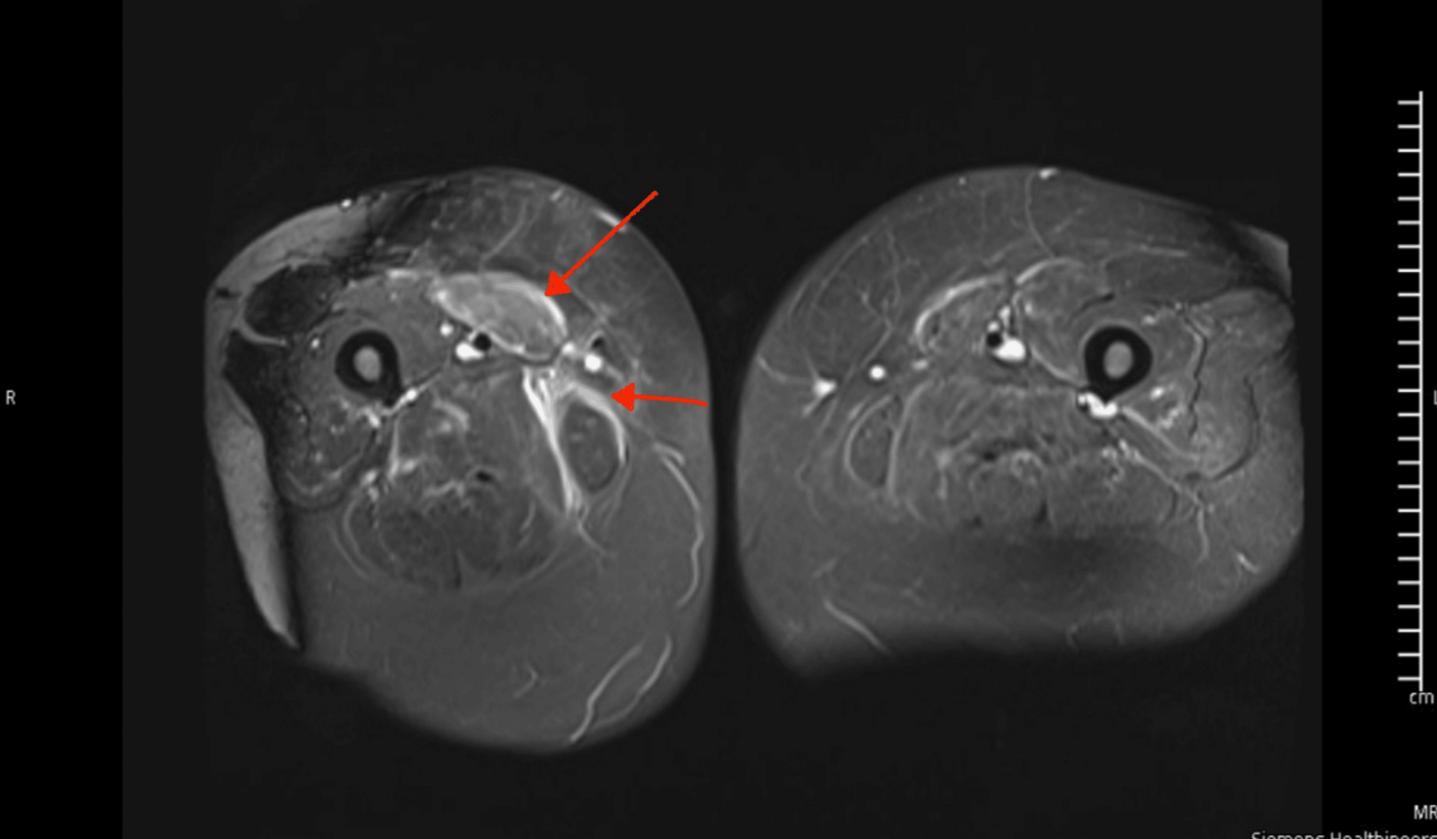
MRI of quadriceps (STIR) showing signal hyperintensities in keeping with muscle inflammation and oedema (myositis) STIR: Short tau inversion recovery
